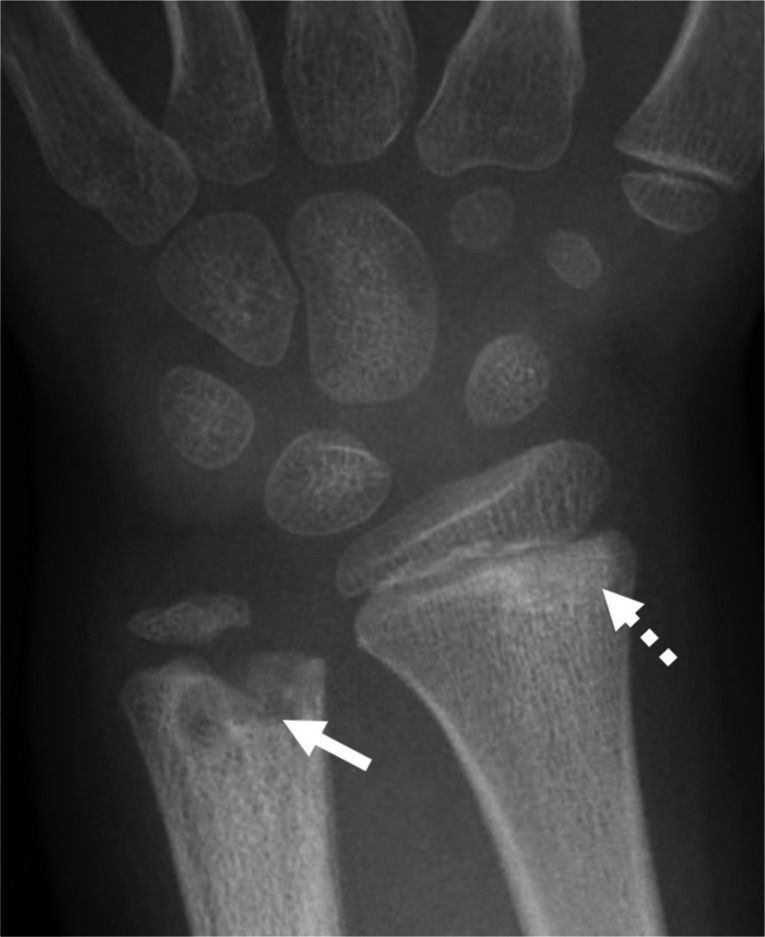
Chronic non-bacterial osteomyelitis (CNO). A 5-year-old girl presented with left wrist pain. The frontal radiograph of the left wrist shows a lytic lesion in the distal left ulnar metaphysis with adjacent sclerosis (*solid arrow*). A more subtle lesion is also present in the distal radial metaphysis (*dashed arrow*). The multifocality and metaphyseal location are suggestive of CNO which was later confirmed
